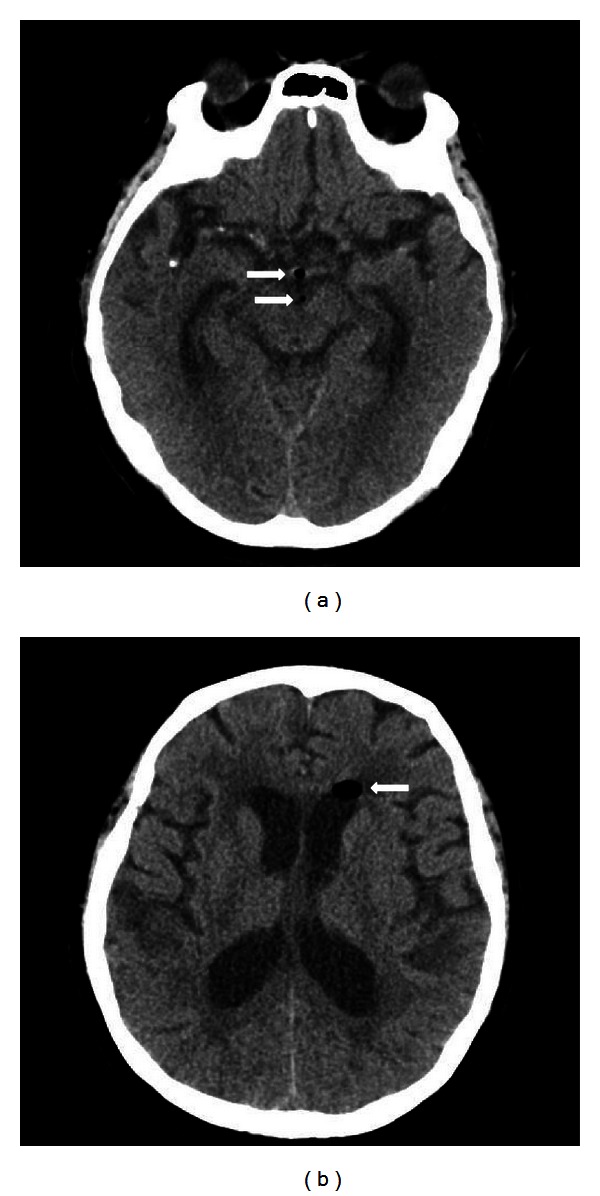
Axial computed tomography (CT) scan of head demonstrated free air (arrows) in the left lateral ventricle frontal horn and basal cistern.
